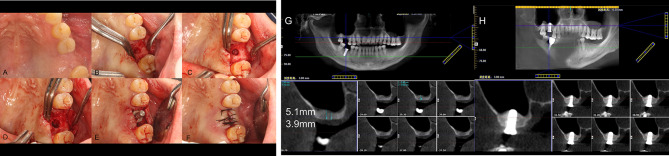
Surgical procedure for modified internal sinus elevation. (A) Preparation for surgery. (B) The alveolar crest is flat and slightly bluish. (C) Bone block serving as the roof of the area of elevated maxillary sinus floor. (D) Separation of the maxillary sinus floor membrane from the alveolar crest using a mucoperiosteal stripper. (E) Implant placement implant (the bone condensing technique was used to obtain good initial stability). (F) Tight suturing after implantation. (G) CBCT before surgery. (H) CBCT immediately after surgery
